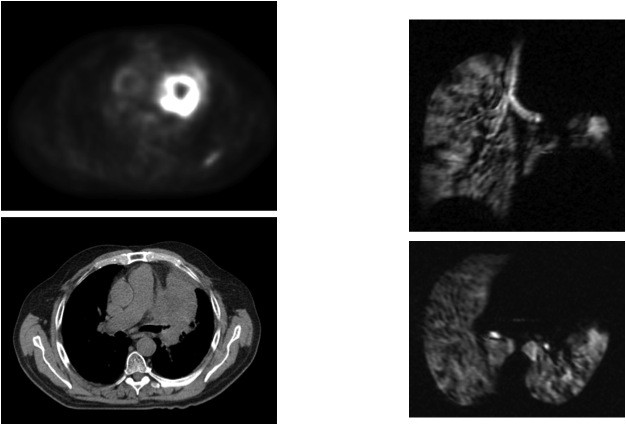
Patient #2 – obstructive left upper lobe mass with complete loss of ventilation on Helium MRI.
